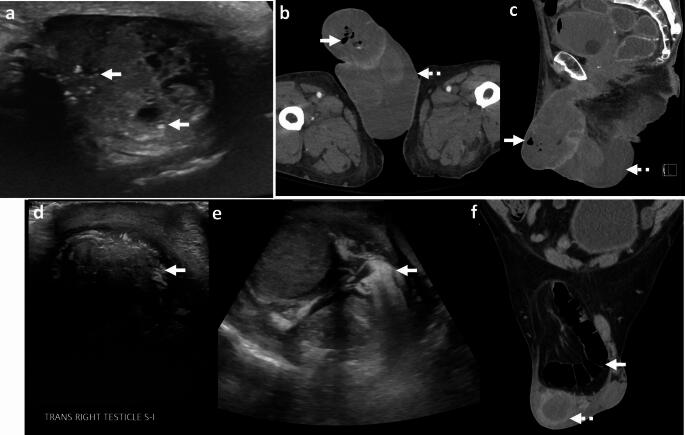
Pitfalls for emphysematous epididymo-orchitis. a Intratesticular echogenic foci: A 28-year-old with right testicular lump. US shows heterogenous mass with necrosis and punctate echogenic foci due to calcifications (solid white arrows, a). Pathology showed yolk sac tumor. b, c Necrotic tumor. A 65-year-old with penile cancer with air foci (solid white arrow, b, c) due to post-radiation tissue necrosis and scrotal wall edema (dotted white arrows, b, c). d–f Inguinoscrotal hernia. A 54-year-old with right scrotal erythema and swelling. Transverse US images show air foci with dirty posterior acoustic shadowing in the right scrotum (solid white arrows, d, e) and a fluid collection (not shown). CT image shows right inguinoscrotal hernia containing colon (solid white arrow, f) and incidental abscess in right hemiscrotum (dotted white arrow, f). The air foci seen on US were due to herniated bowel and were not related to the incidental scrotal abscess
